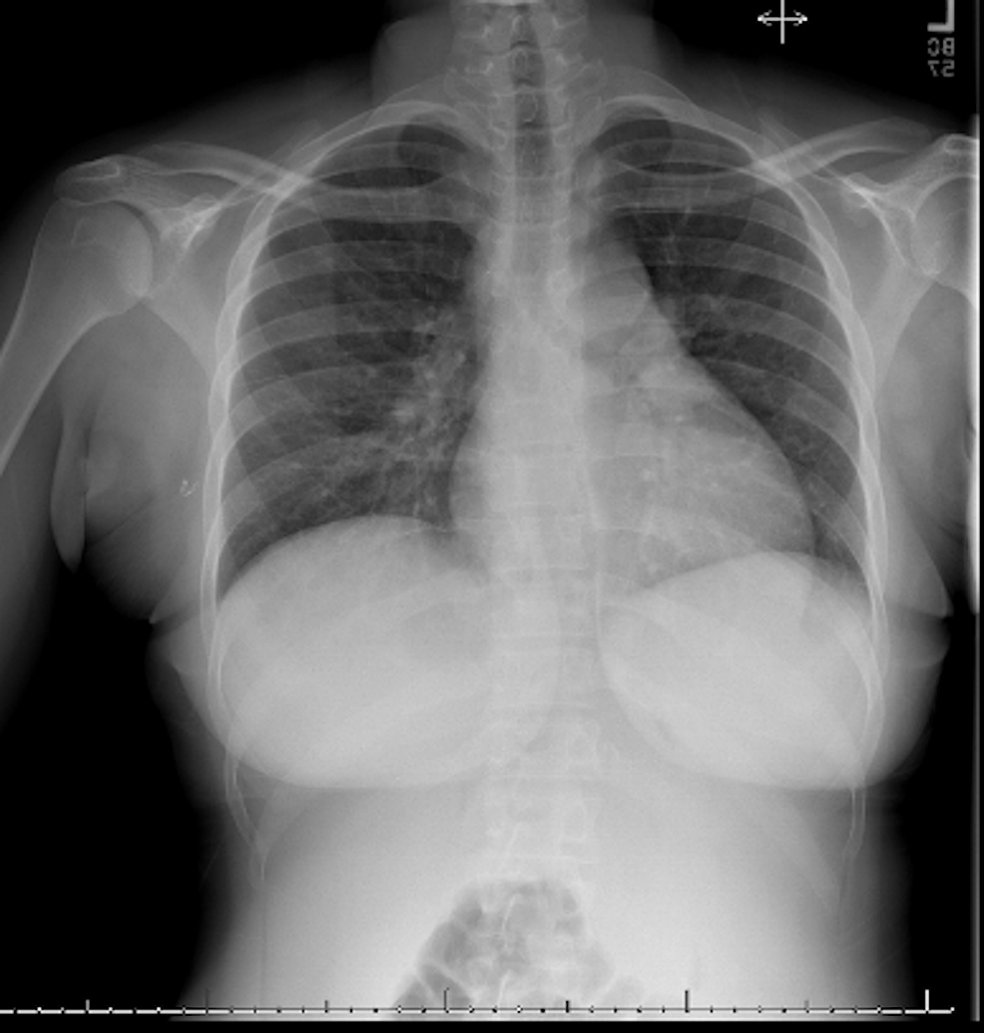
Cardiomegaly on chest x-ray
